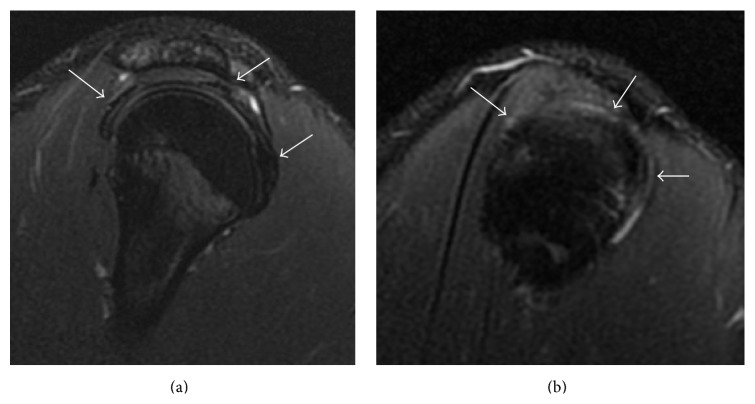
Sagittal T2 FS SE MR images of the right shoulder (TR = 2600; TE = 63.9; NEX = 3; EC = 1) (a) and (b) demonstrating the rotator cuff tendons (white arrows) with normal thickness and hypointensity, without tears.
